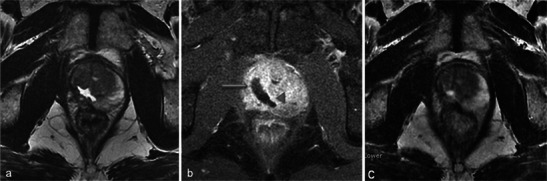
Retreatment of the same lobe. A 60-year-old patient with retreatment of the right lobe. Day-7 post-retreatment axial T2-weighted MR image (a) and axial T1 FS contrast-enhanced MR image (b) show a slight volume increase of the treated lobe compared with the first procedure; the area of prostate necrosis presents two different intensity levels: the residual fluid cavity (*arrowhead*) and tissue necrosis (*arrow*), with less low intensity. Note, 6 months post first treatment axial T2-weighted MR image (c) corresponding to pre-retreatment aspect
